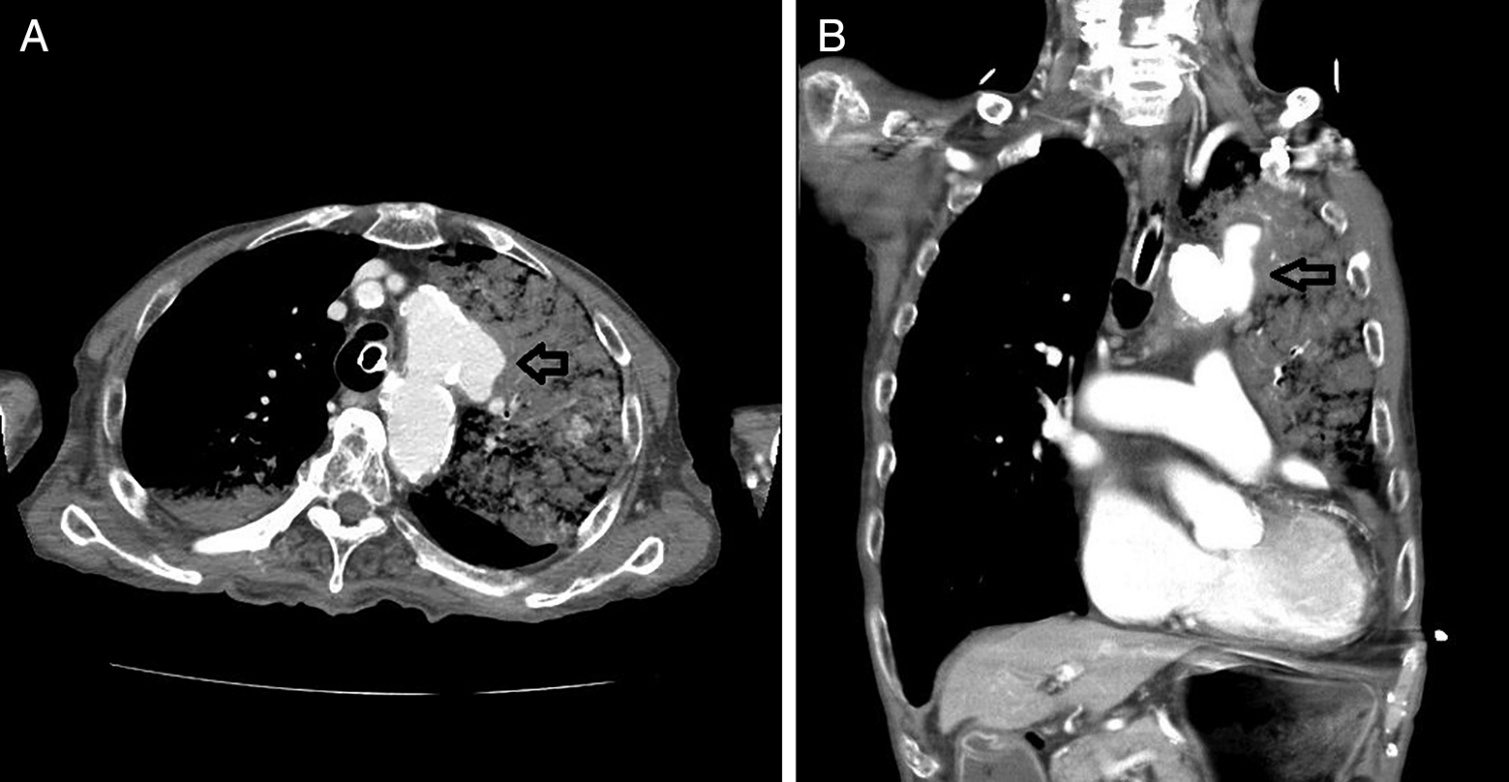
Contrast computed tomography showed an aortic aneurysm at the aortic arch that penetrated the upper lobe of the left lung; blood flowed into the upper lobe of the left lung. (A) Axial view; (B) coronal view.
